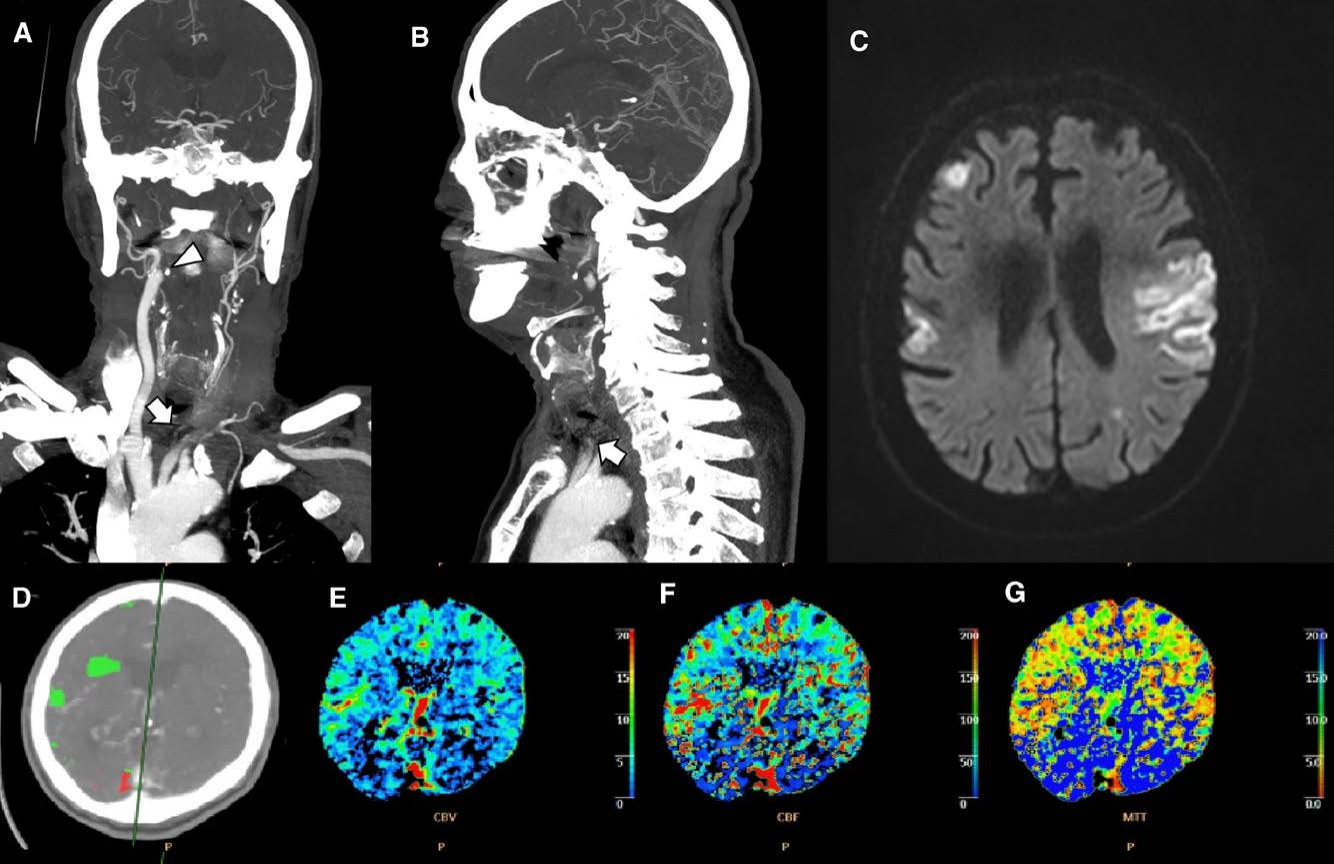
Left middle cerebral artery territory infarction with left CCA and right ICA occlusion (Case 3). (A) Left common carotid artery (CCA, arrow) and right proximal ICA occlusion (arrowhead) in coronal computed tomography angiography (CTA) imaging. (B) Left CCA occlusion (arrow) in sagittal CTA imaging. (C) There is multifocal infarction in the bilateral parietal and frontal lobes on (D–G) diffusion-weighted magnetic resonance imaging. Perfusion CTA images show increased mean transit time and decreased cerebral blood flow and cerebral blood volume in the posterior circulation. CCA = common carotid artery, ICA = internal carotid artery.
